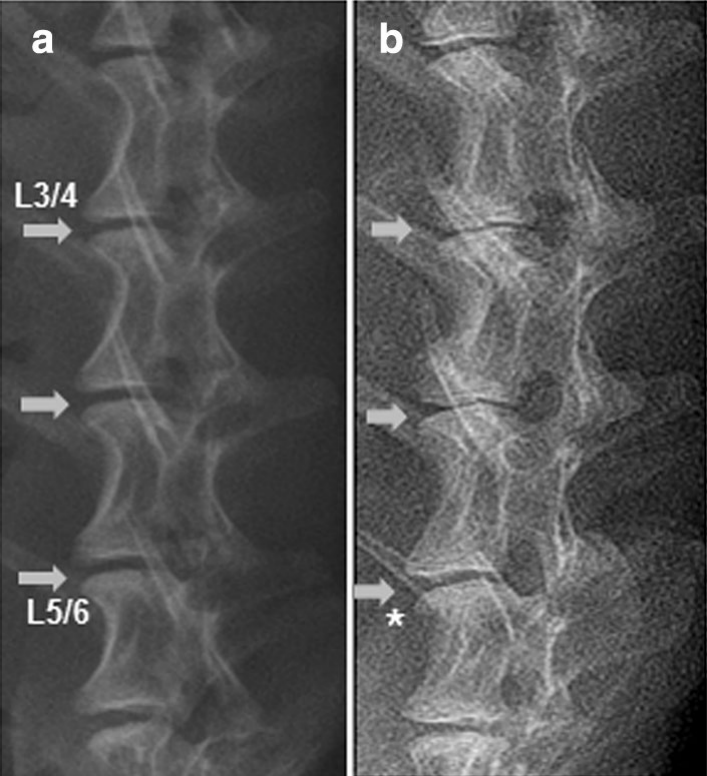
Representative lateral radiographs before surgery (a) and 6 months after surgery (b). Three experimental levels showed obvious and various degrees of disc space narrowing after surgery. Osteophyte formation (*white asterisk*) was seen at the margin of the vertebral bodies. The *arrows* indicate the operated disc
